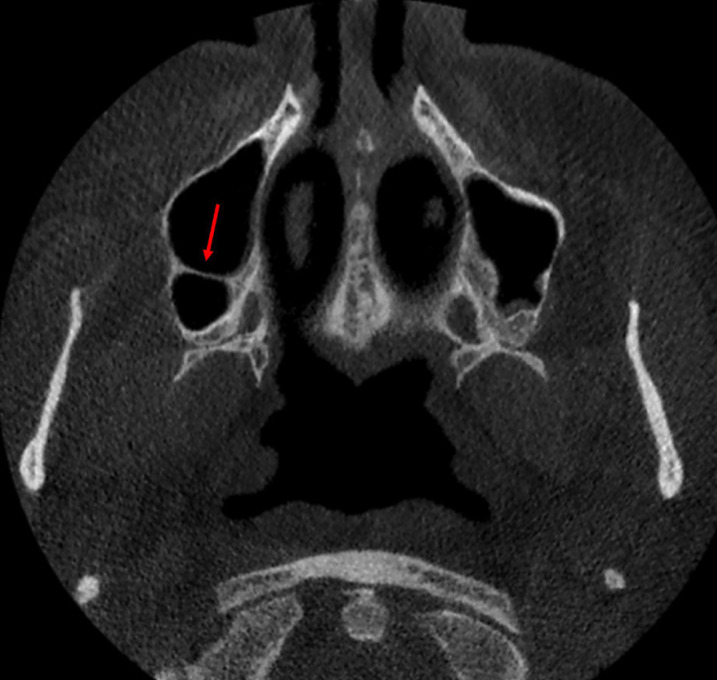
Axial view of cone-beam computed tomography showing a complete septum in the posterior area of the right maxillary sinus.
